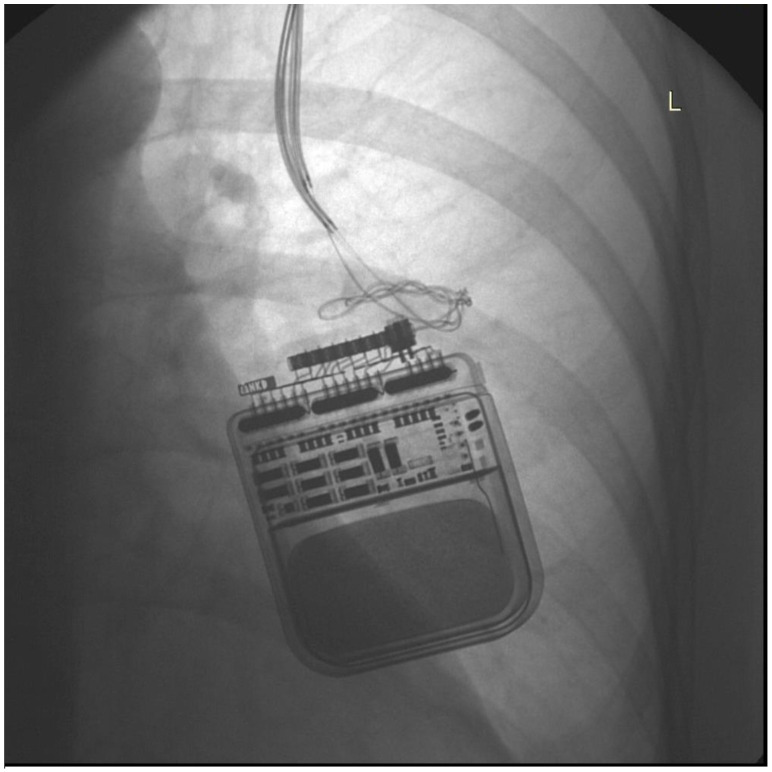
Chest X-ray showing IPG and coiling of extension wires.
